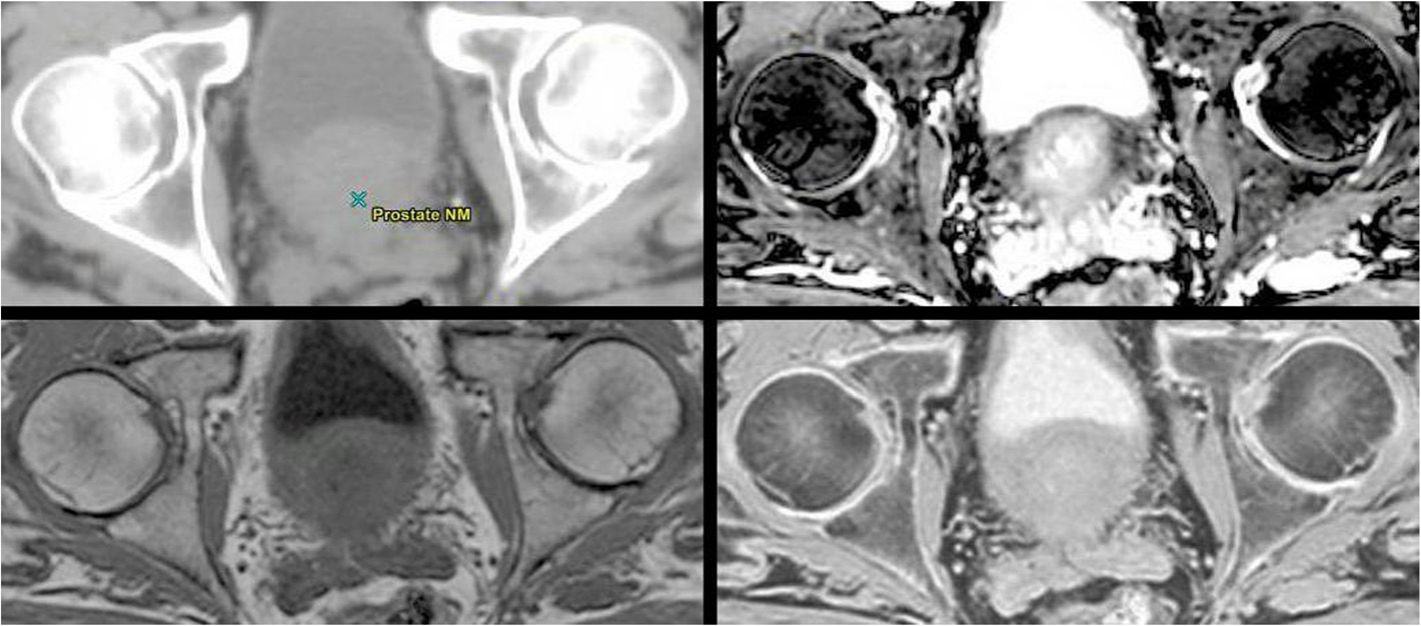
Different imaging sequences comparing bone boundaries. Detailed Legend: Imaging sequence from top left going clockwise. CT, bTFE, Inverted T1, T1. Notice that boundary of the femoral heads and other bony tissue shows up as a region of no signal in the bTFE and T1 image. On the CT and the inverted T1 image, this edge is readily identifiable.
